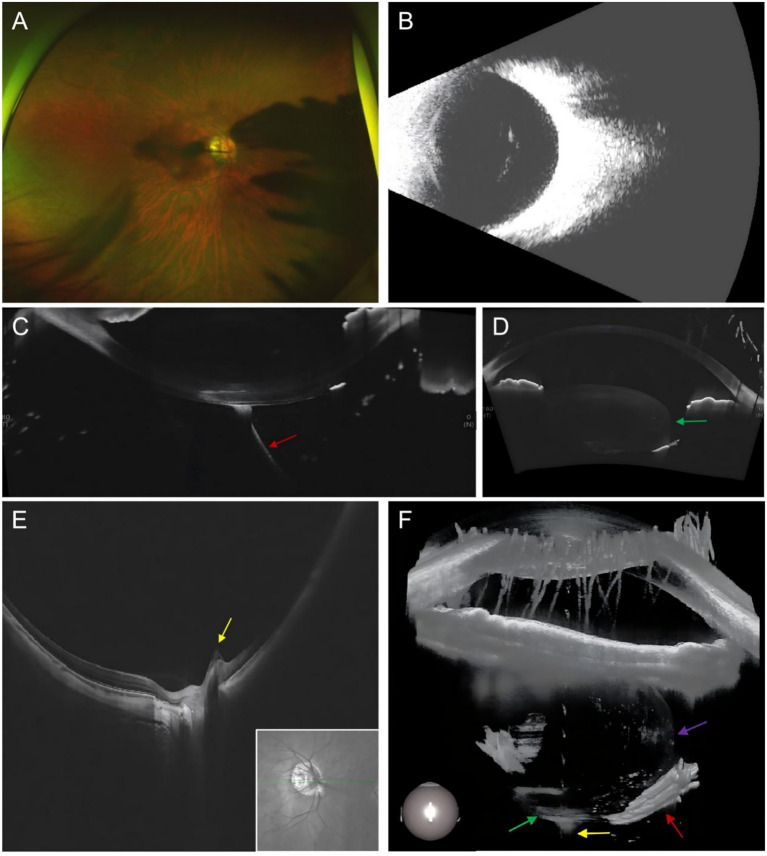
Preoperative ocular examinations. (A) Preoperative SLO examination revealed posterior pole obscuration, peripheral opacities, tigroid-patterned appearance and peripapillary atrophy; (B) preoperative B-scan ultrasonography showed elongated axial length and vitreous floaters; (C) AS-OCT showed a band-shaped high reflectivity signal on the central posterior capsule, extending into the vitreous cavity (red arrow); (D) AS-OCT showed regional lens defect and zonular defect on nasal equatorial area (green arrow); (E) optic disc OCT revealed a localized short linear medium-intensity signal in the optic disc region (yellow arrow); (F) three-dimensional AS-OCT showed pigmented membrane tissue attached to the nasal side of the posterior capsule (red arrow), opacification at the posterior pole of the lens (green arrow), residual primary vitreous adherent posterior to the posterior capsule (yellow arrow), and regional lens defect and zonular defect on on nasal equatorial area (purple arrow).
